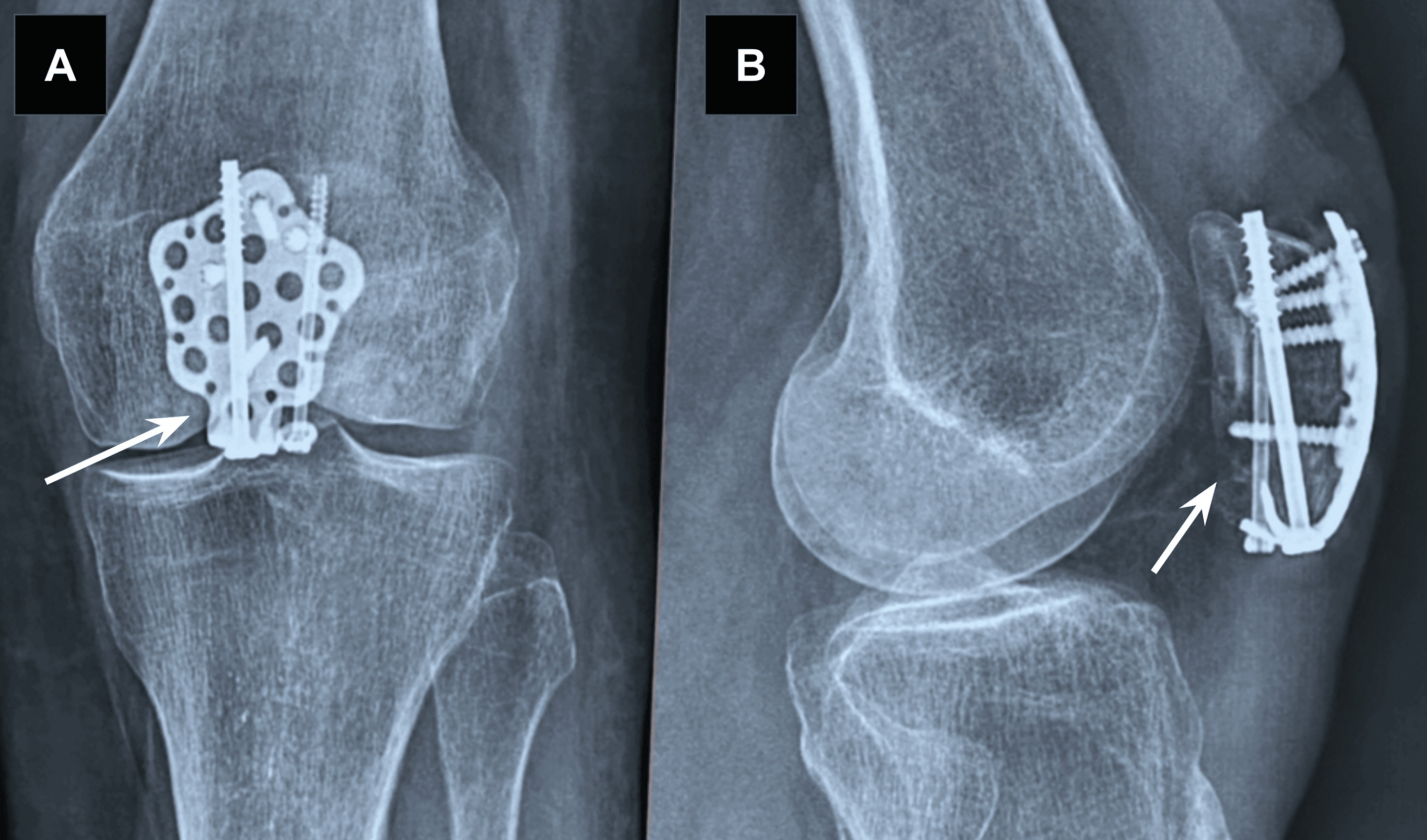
Left knee—anteroposterior (A) and lateral (B) radiographs indicating complete osseous union and no wound complications at postoperative Week 23.
